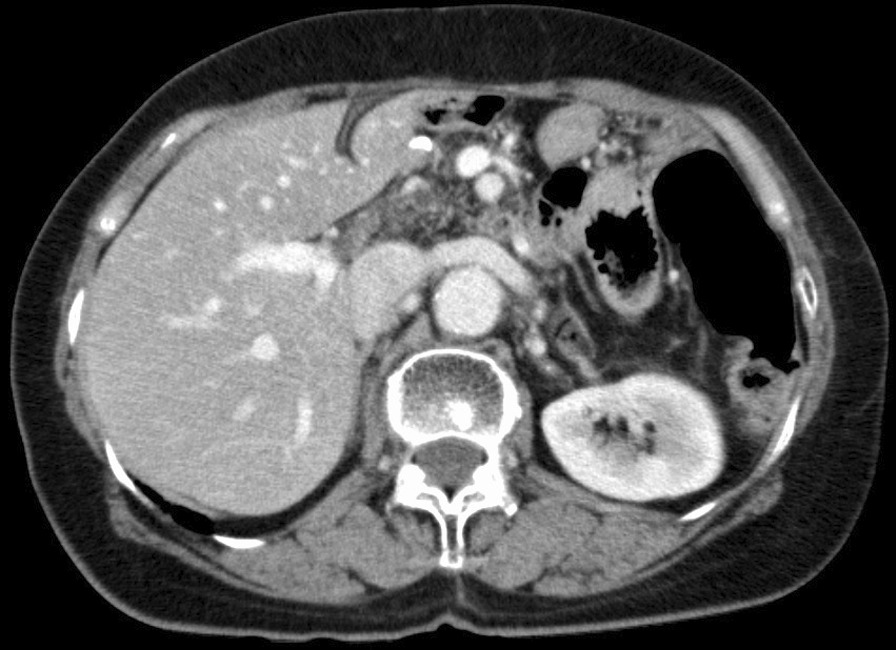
Computed tomography (coronal plane). No nodules can be identified
